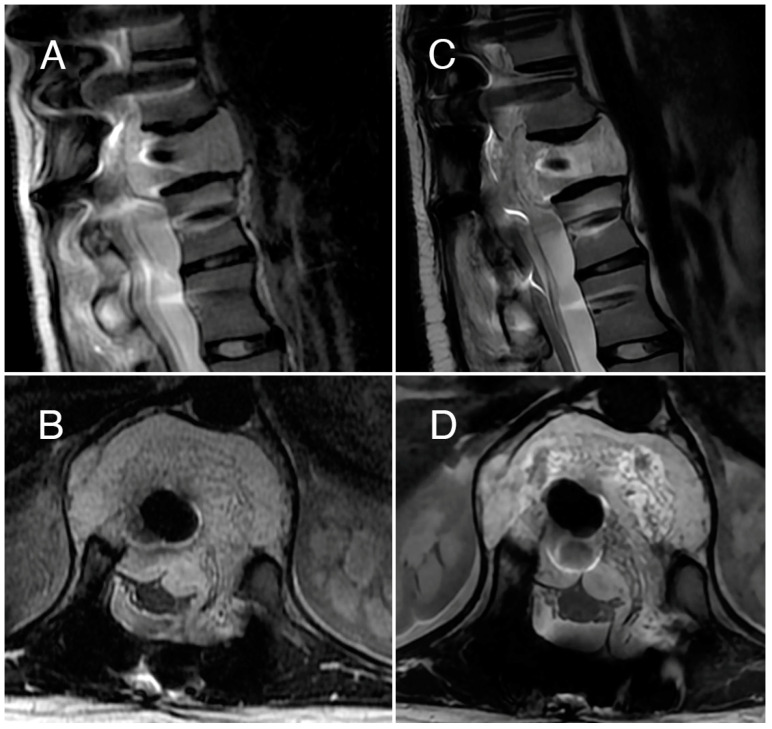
Case 2: T2-weighted MRI scans performed before (A,B) and 7 months after the ECT treatment (C,D). Imaging from before the treatment indicates a significant spinal cord compression at T12 level, which appears to be reduced after ECT treatment.
